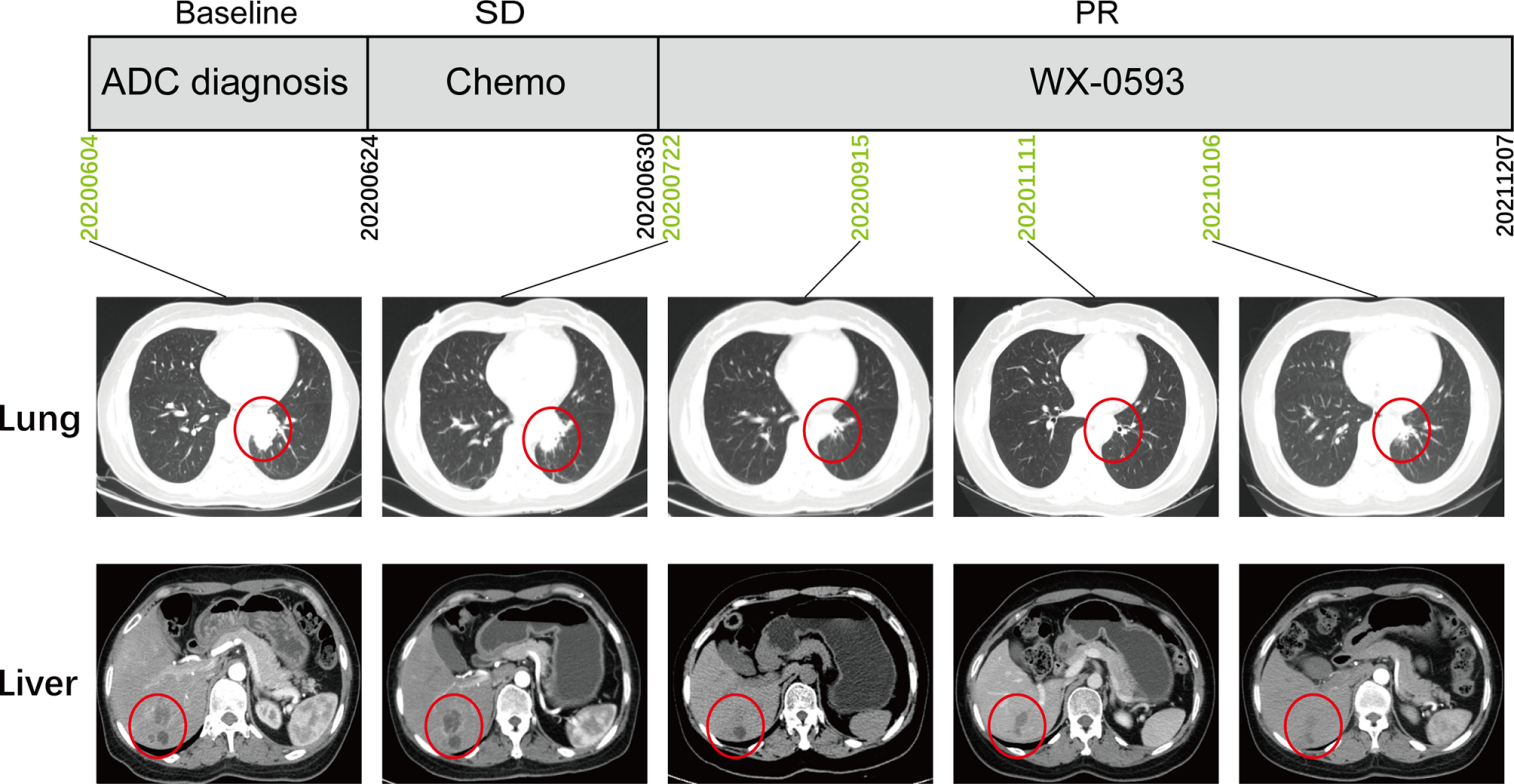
The treatment scheme and representative CT scan images during treatment. ADC, adenocarcinoma; SD, stable disease; PR, partial response.
